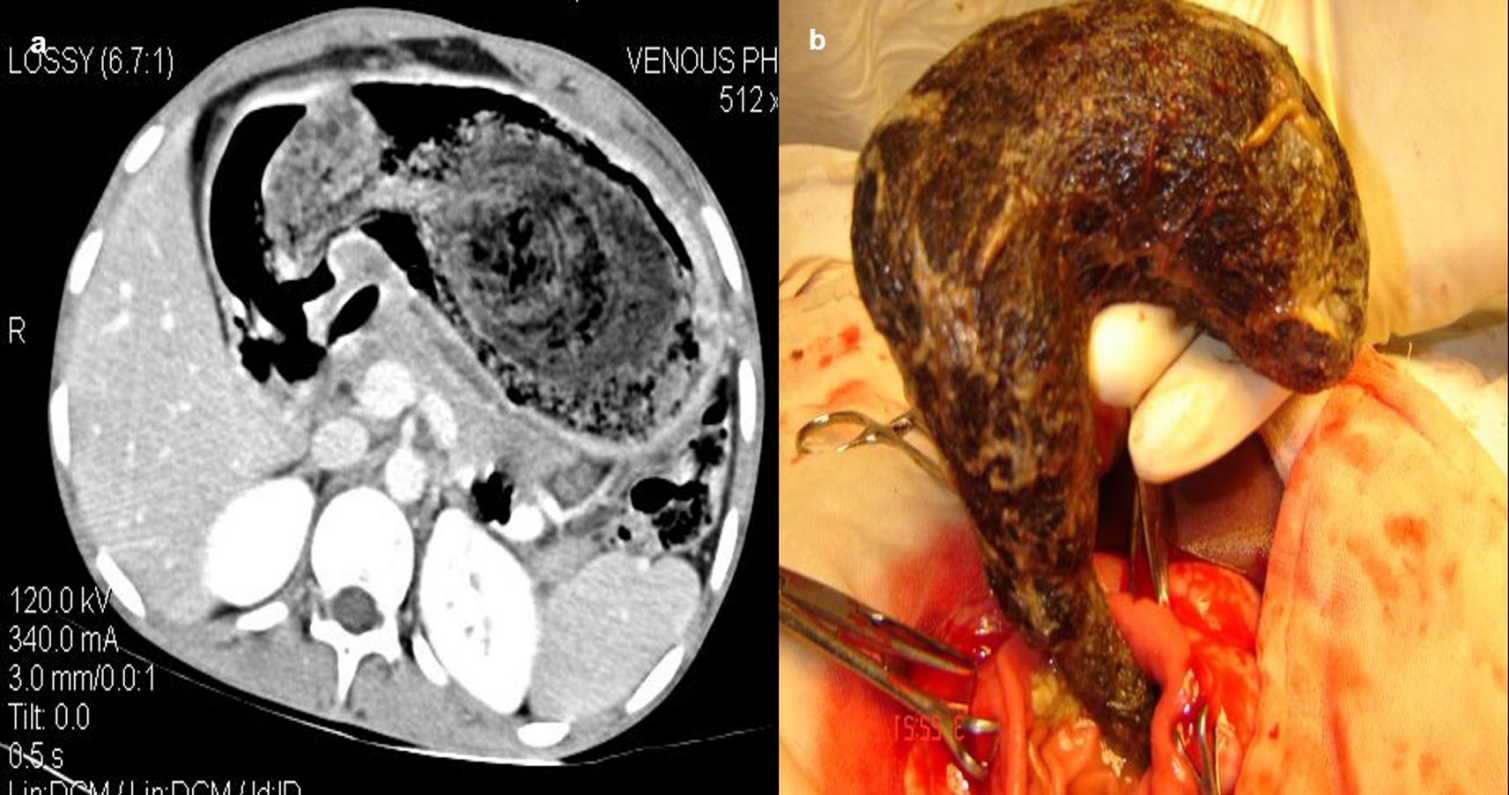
a & b: Contrast enhanced Computed tomography (CECT) axial section reveals a heterogeneous lamellated non-enhancing soft tissue density mass intraluminally in the stomach, conforming to its shape and extending into the antrum and pylorus -trichobezoar (a). Gastrotomy revealed the ball of hair in the stomach (b).
